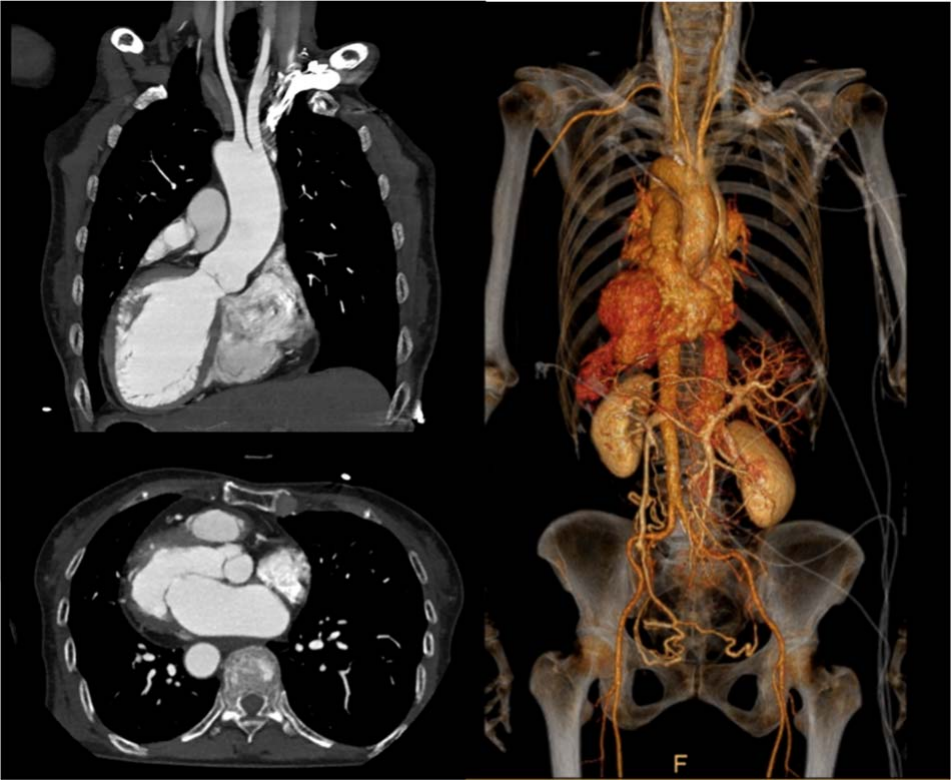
Preoperative contrast-enhanced CT: axial, coronal oblique MIP, and coronal oblique 3D VR images demonstrating situs inversus totalis and dextrocardia.
